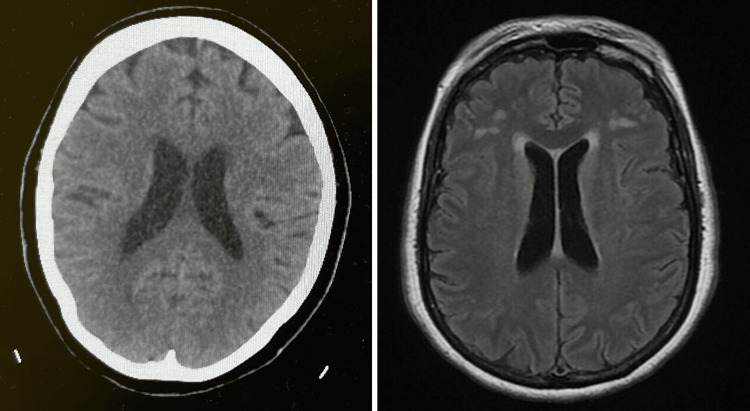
Head CT (left): Periventricular and deep white matter hypodensities, providing clinically significant indicators of chronic ischemic changes associated with small vessel disease. MRI brain scan with intravenous contrast (right): mild periventricular white matter diffusion, variable-sized lesions, hyperintense signals on FLAIR and T2W images. Findings suggest chronic ischemic infarcts and small vessel disease. Mild ventricular dilatation and widening CSF spaces are noted. FLAIR: fluid-attenuated inversion recovery; T2W: T2-weighted
